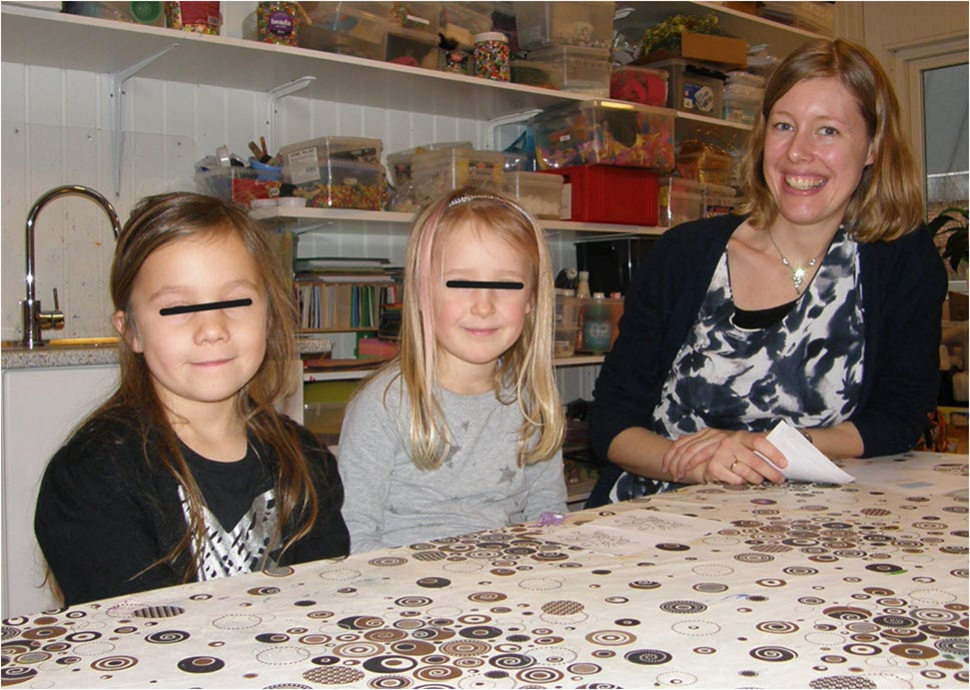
Pilot testing the visual analogue scale for evaluating comfort of children during magnetic resonance imaging in a Danish kindergarten (2016). Published with written consent from parents. Photo: Lotte Blanner
